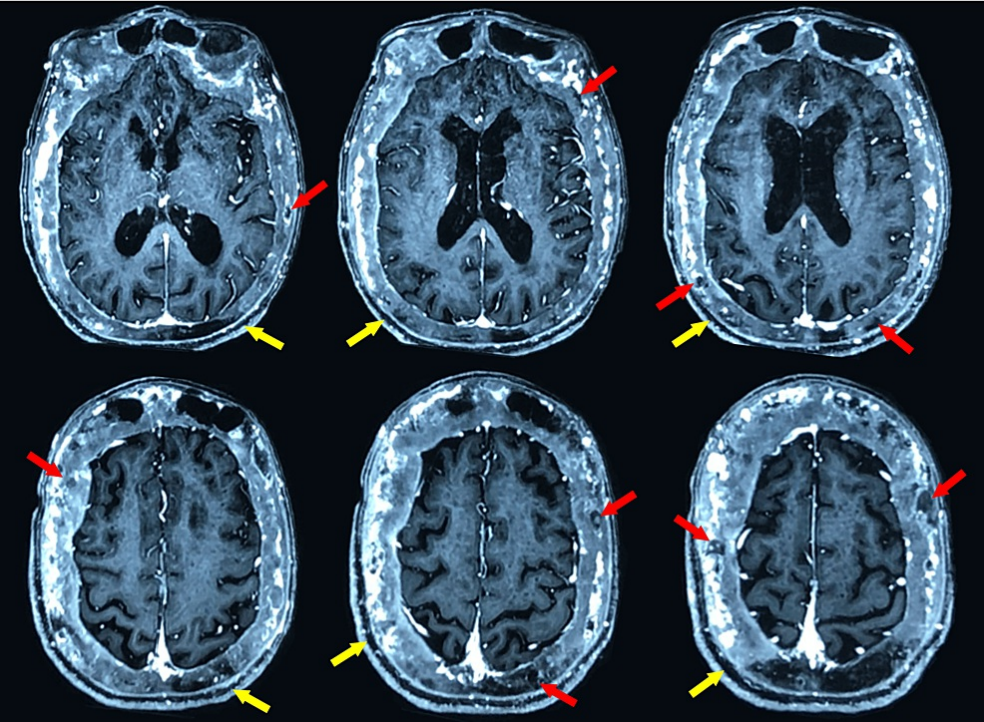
Axial T1-weighted post gadolinium brain MRI Osteoporosis circumscripta of the skull with large, lytic lesions (red arrows), a widened diploic space (yellow arrows), and right frontal lobe compression by bone thickening.
